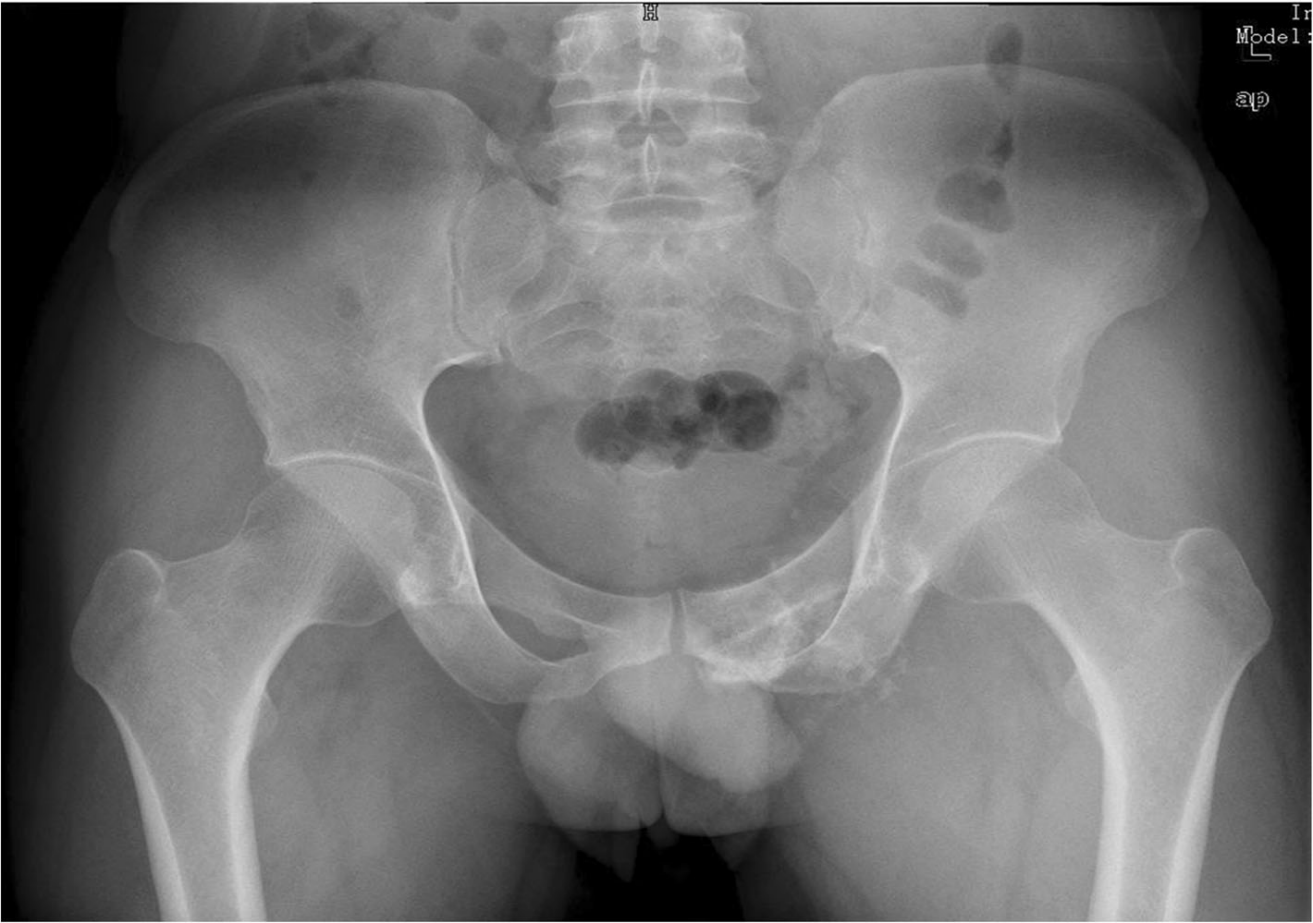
Pelvic X-ray in a patient with a chondrosarcoma (after biopsy) involving the superior and inferior pubis
